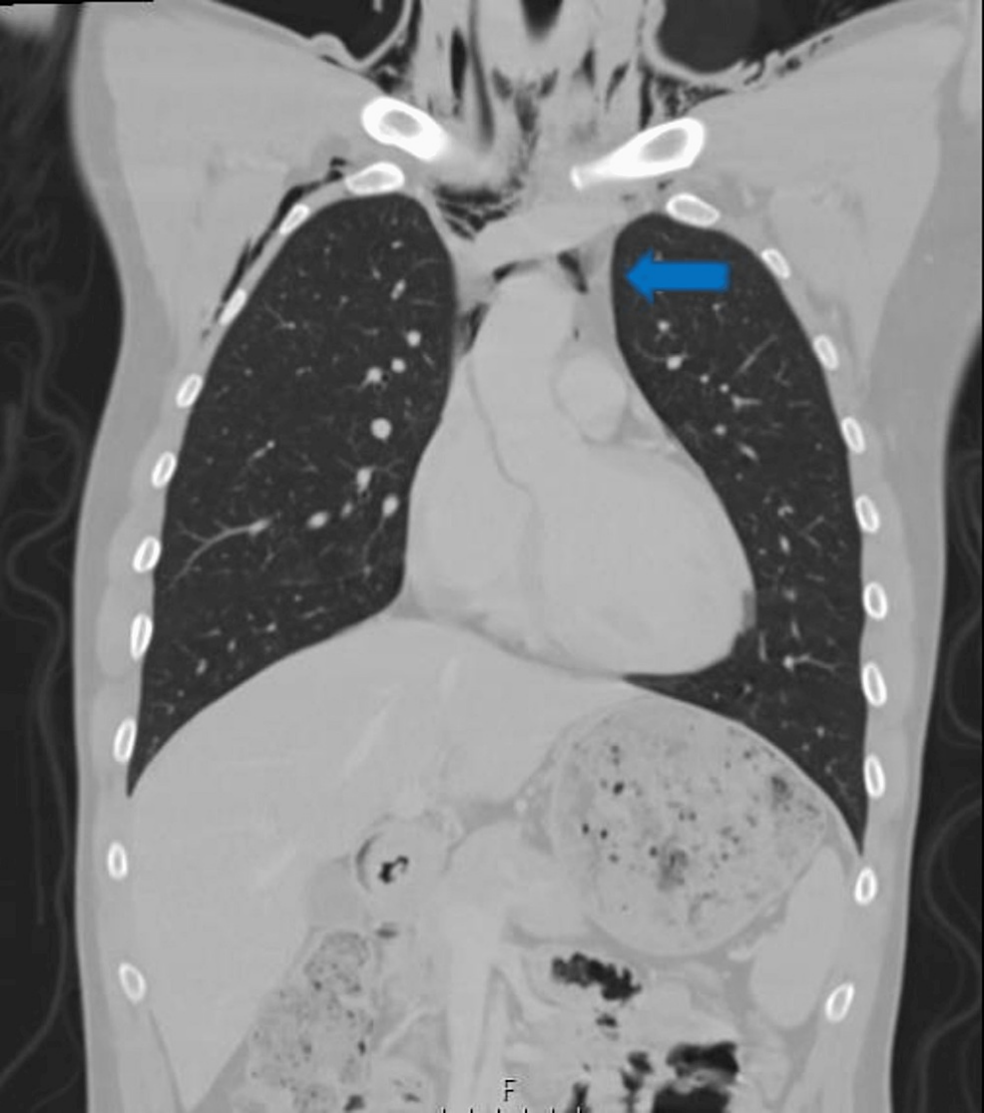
Admission thoracic CT: Pneumomediastinum
